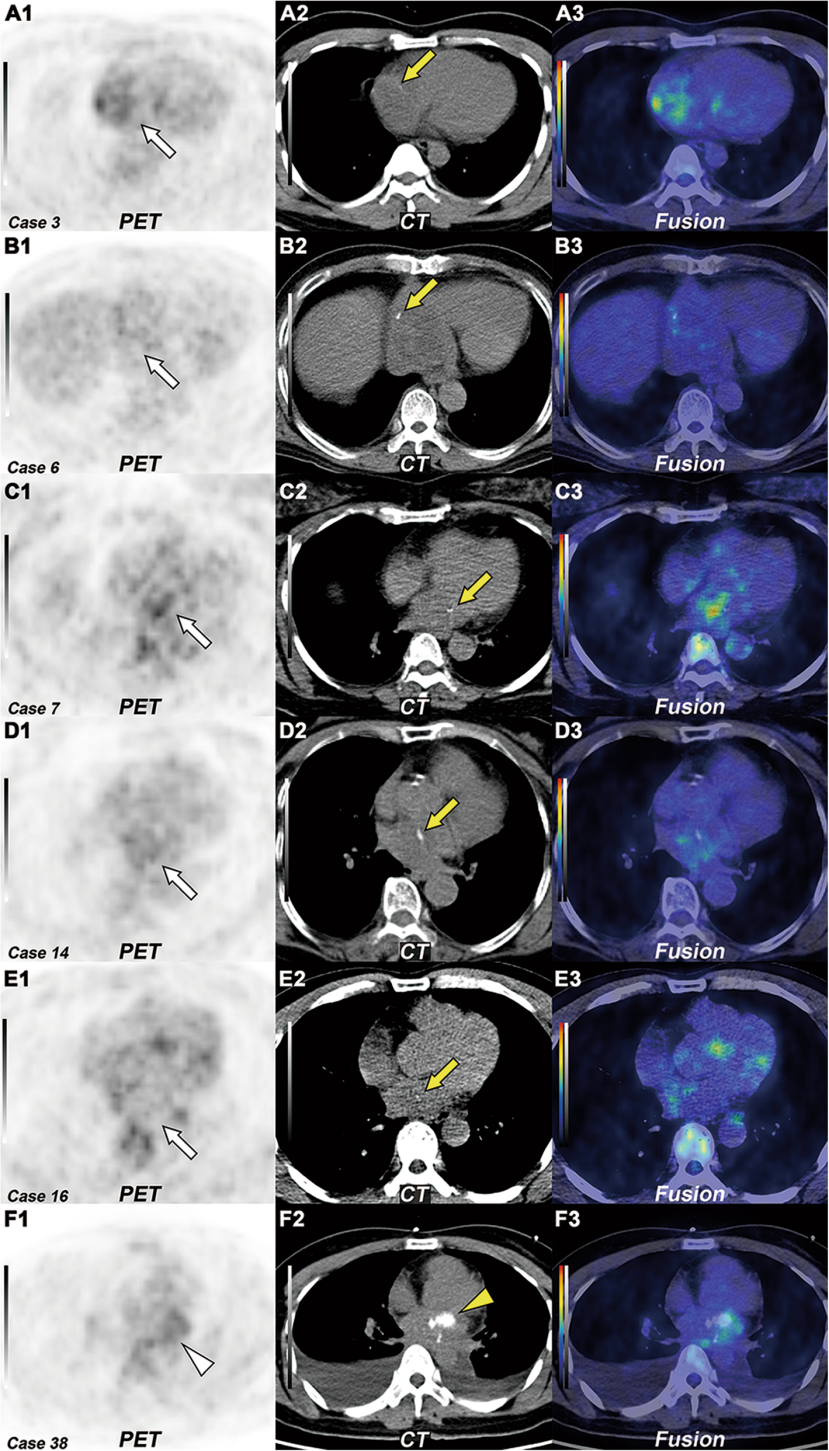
Intratumoral calcification in myxoma and myxosarcoma. A–E The transverse view of selected PET (1), CT (2), and fused PET/CT (3) images revealed five myxomas with none to mildly increased ^18^F-FDG uptake (white arrow, SUVmax 2.2–4.7). On the non-contrast CT (A2–E2), the tumor presented punctate calcification (yellow arrow). F1–F3 The transverse view of selected PET, CT, and fused PET/CT images revealed a myxosarcoma with mildly increased ^18^F-FDG uptake (white arrowhead, SUVmax 3.5). On the non-contrast CT (F2), the tumor presented nodular calcification (yellow arrowhead)
